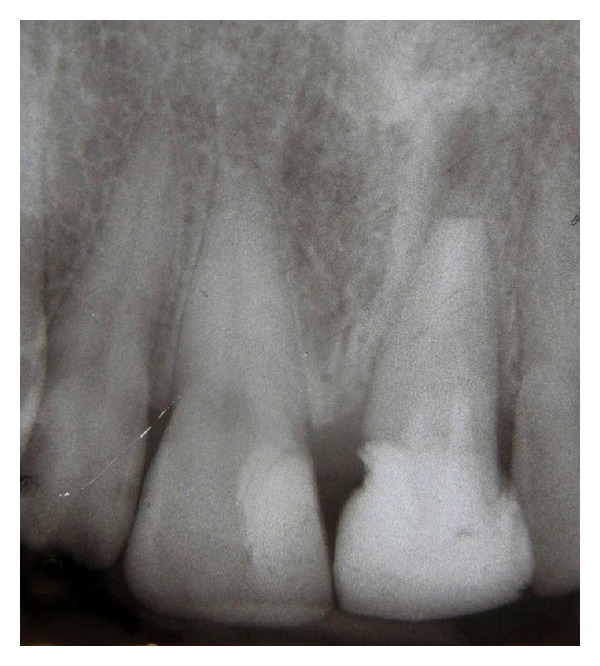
12 months after intentional replantation; periapical radiolucency was noticeably reduced.
